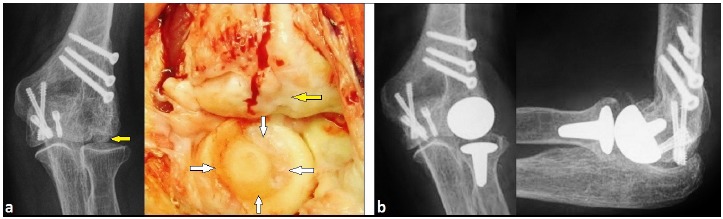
(Case Presentation): (a) PA radiograph and intraoperative clinical photograph four years after ORIF including one year after partial removal of headless compression screws showing distinctive OA of the capitellum (yellow arrows) without any signs of degenerative changes of radial head radiographically, but intraoperatively there was pronounced deep circular cartilage defect (white arrows). (b) PA and lateral radiographs showing correct positioning and alignment of LRE™ system without any signs of overstuffing.
